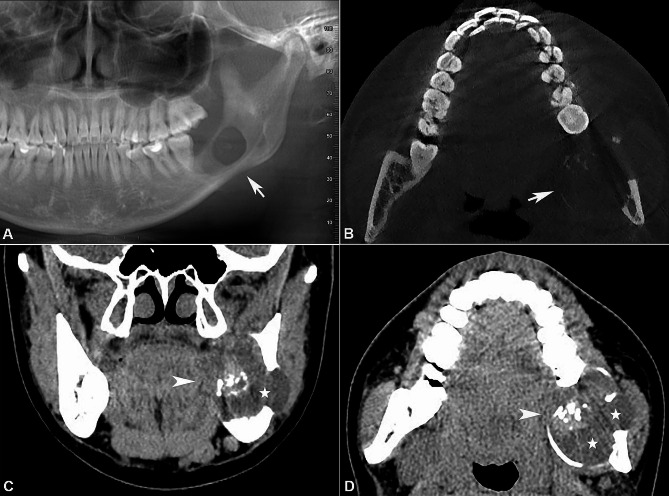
Radiographic findings. Reconstructed panoramic view (A) and axial view (B) of CBCT images showed a unilocular, well-defined radiolucency with radiopaque materials in the left posterior mandible, with significant expansion and perforation of the buccal and lingual cortical plates (arrow). Coronal view (C) and axial view (D) of MDCT images with soft tissue algorithm demonstrated a well-delineated, heterogeneous mass consisting of solid (arrow head) and cystic (star) areas. Radiopaque calcifications were prominent
